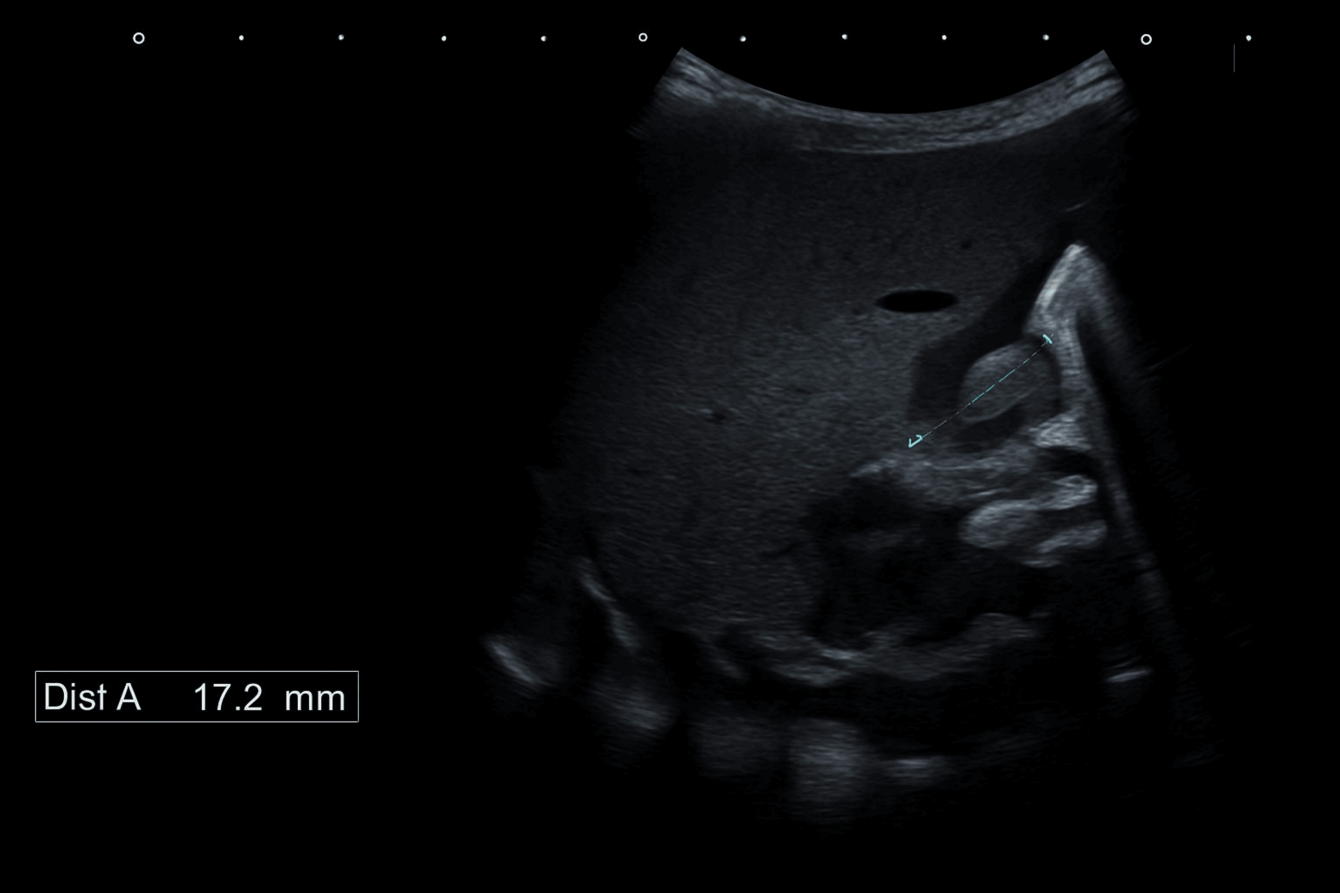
Ultrasonography showed a canal length of 17.2 mm
